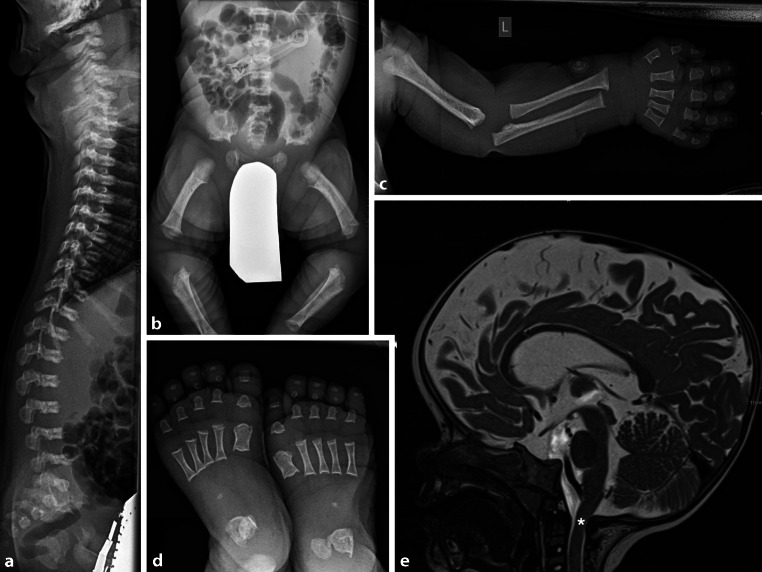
Achondroplasia. a Vertebral bodies with flattening, anterior beaking and thoracolumbar kyphosis. b Dysmorphic pelvis. c Rhizomelia and trident hand. d Dysmorphic metatarsals and phalanges. e MRI with foramen magnum stenosis (*asterisk*)
